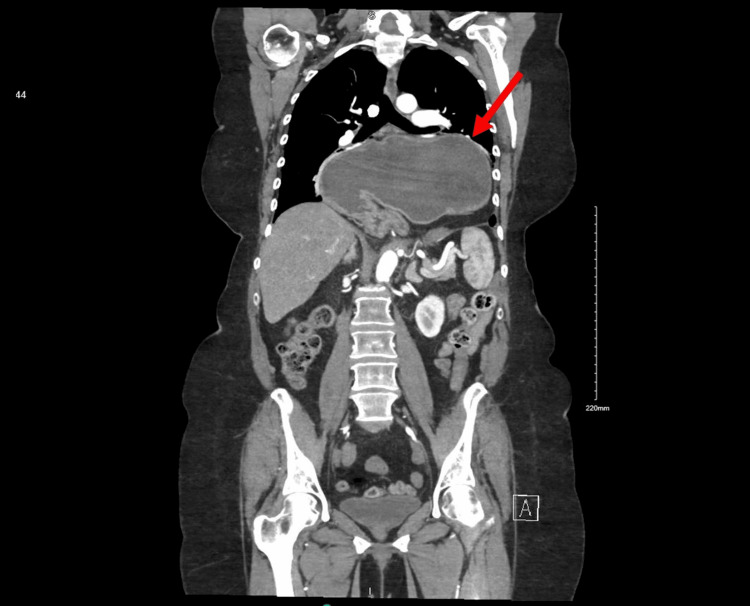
CT angiography of the chest taken on hospital day 1 The image demonstrated a very large posterior diaphragmatic hernia with the stomach extending into the mid chest level. An organoaxial volvulus with the duodenum located inferiorly was also identified CT: computed tomography
